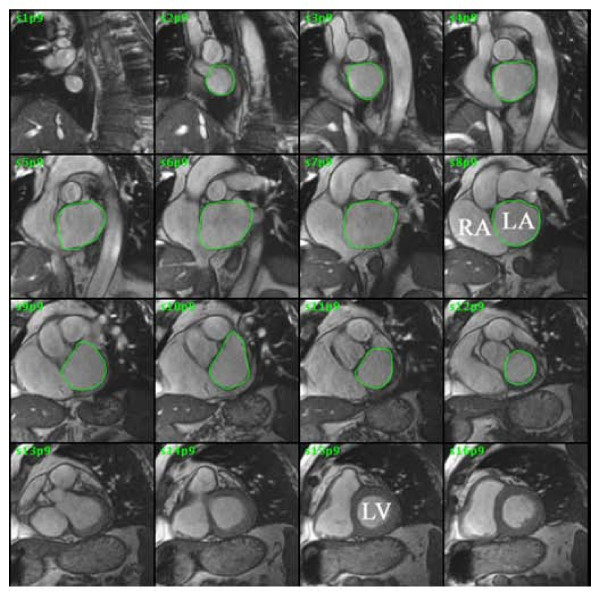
MRI of left atrium. Steady state free precession cine gradient echo was performed on sequential slices of the left atrium. The left atrial border was traced at end-left ventricular systole. The summation of the areas multiplied by slice thickness is the left atrial volume. LA = left atrium, RA = right atrium, LV = left ventricle.
